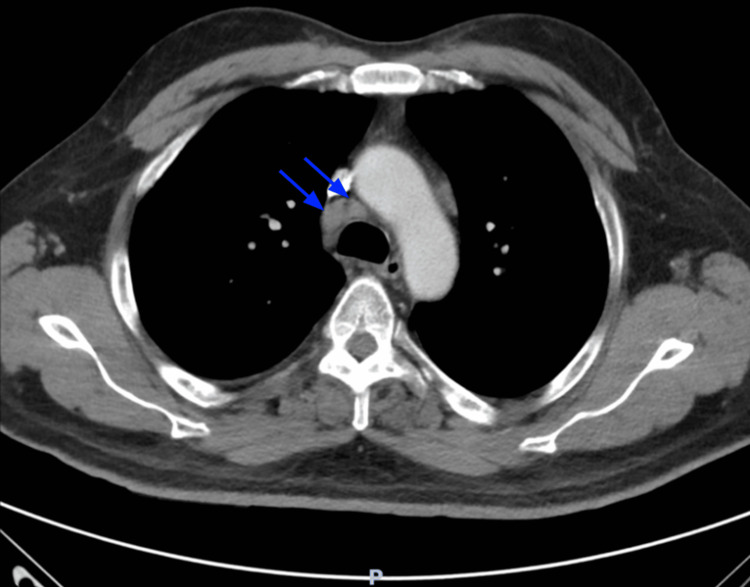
Axial CT chest with intravenous contrast showing 1.1 cm right paratracheal lymph nodes present (blue arrows) CT: computed tomography
